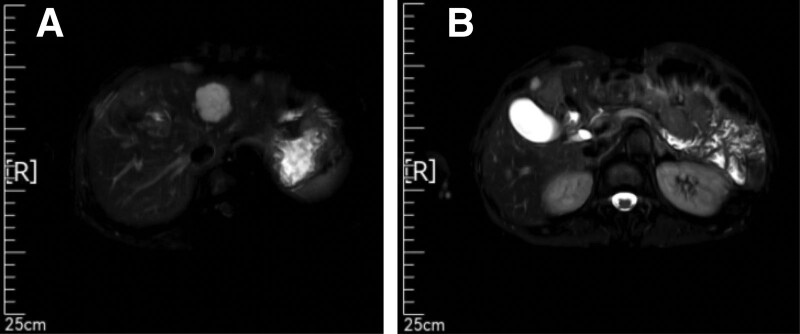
The patient MRI reexamination results on May 8, 2021 showed a mass at the junction of liver S4 and liver S8 segments, with high perfusion of the surrounding liver parenchyma, and enhanced small nodules visible in the hepatic artery phase, indicating possible metastasis. MRI = magnetic resonance imaging.
